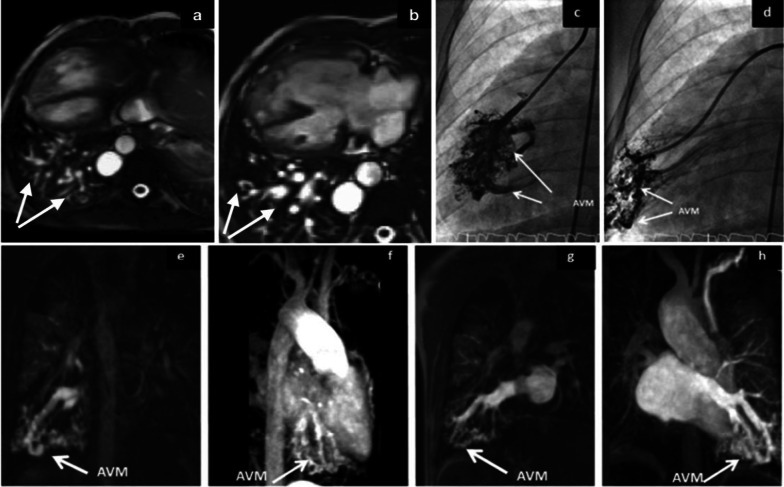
A large right-sided pulmonary AVM in a patient with a Fontan circulation. a, b Axial still shots of MRI SSFP cine sequences demonstrating a pulmonary AVM in the right lower lobe, c, d catheter angiogram confirming the presence of an AVM, e–h contrast-enhanced MRI angiogram in coronal planes demonstrating the AVM (white arrows). AVM: arteriovenous malformation. Re-used with permission from Fontan circulation in an adult: A guide for the radiologist. Arzanauskaite M, Nyktari E, Voges I. ESTI-ESCR 2018 / P-0103
